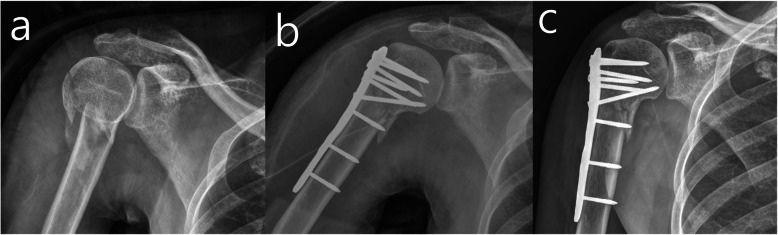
Simple radiographs of the far cortical locking system fixation. a Preoperative, b Postoperative and c 3 months after surgery
